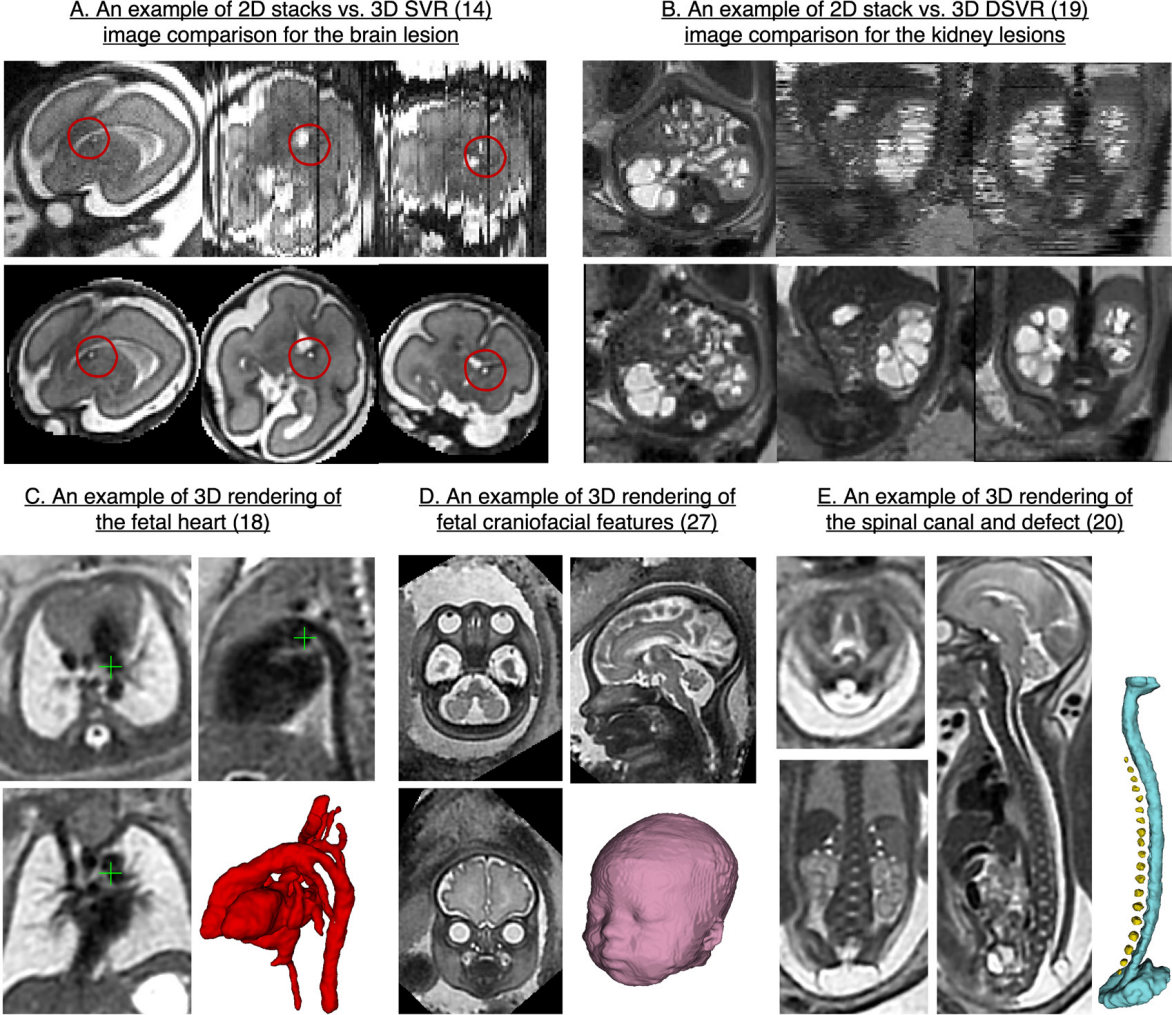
Visualisation of 3D reconstructed images for diagnostic purposes. The examples are based on the MRI datas acquired at St.Thomas’ Hospital, London.
